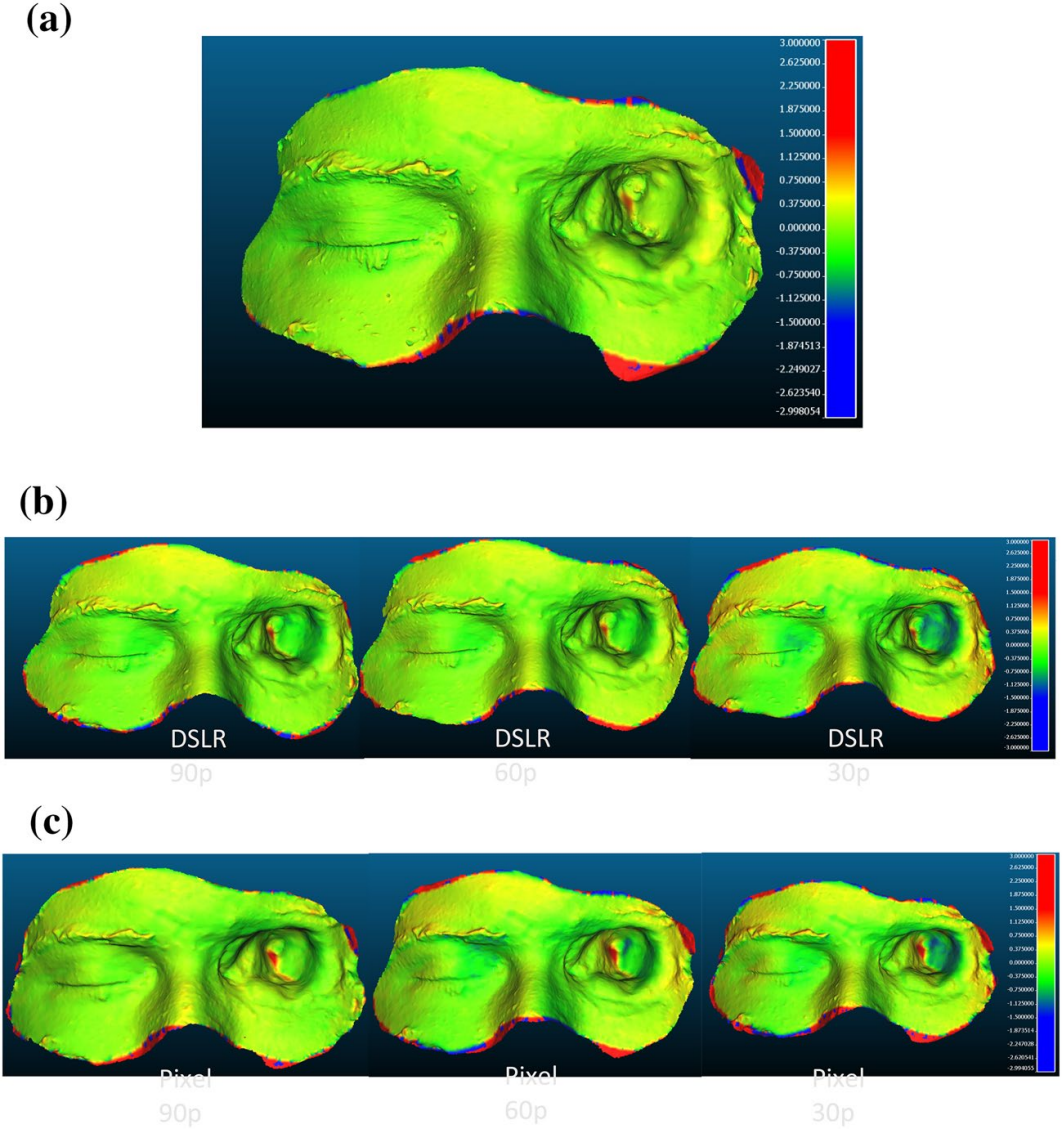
(a) 3D Surface deviation of Comet 3d scanned model (CT scanned model is taken as a Reference model for the analysis). (b) Effect of no. of photos on the 3D Surface deviation of photogrammetry model generated by DSLR data (CT scanned model is taken as a Reference model for the analysis. (c) Effect of no. of photos on the 3D Surface deviation of photogrammetry model generated by Google Pixel Smartphone data (CT scanned model is taken as a Reference model for the analysis.
